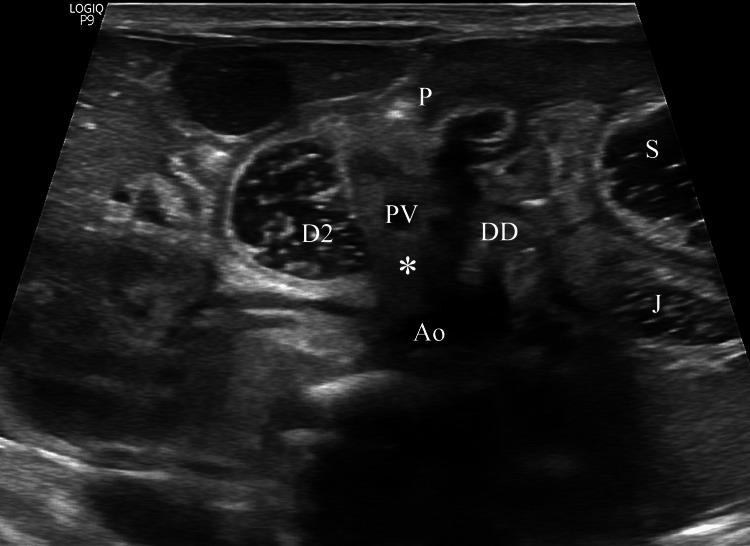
Saline-aided ultrasound image showing an S-shaped formation of dilated and nondilated duodenal segments, along with hyperechogenic tissue at the transition point The gray-scale midline image shows marked dilation of the stomach and descending duodenum with saline, whereas the nondilated distal duodenum crosses the midline anterior to the aorta. The dilated and nondilated segments of the duodenum form an S-shape. A hyperechogenic tissue is recognized (asterisk) anteriorly to the aorta, likely arising from the pancreas and enveloping the horizontal duodenum. Ao, Aorta; D2, Descending duodenum; DD, Distal duodenum; J, Jejunum; PV, Portal vein; P, Pylorus; S, Stomach
